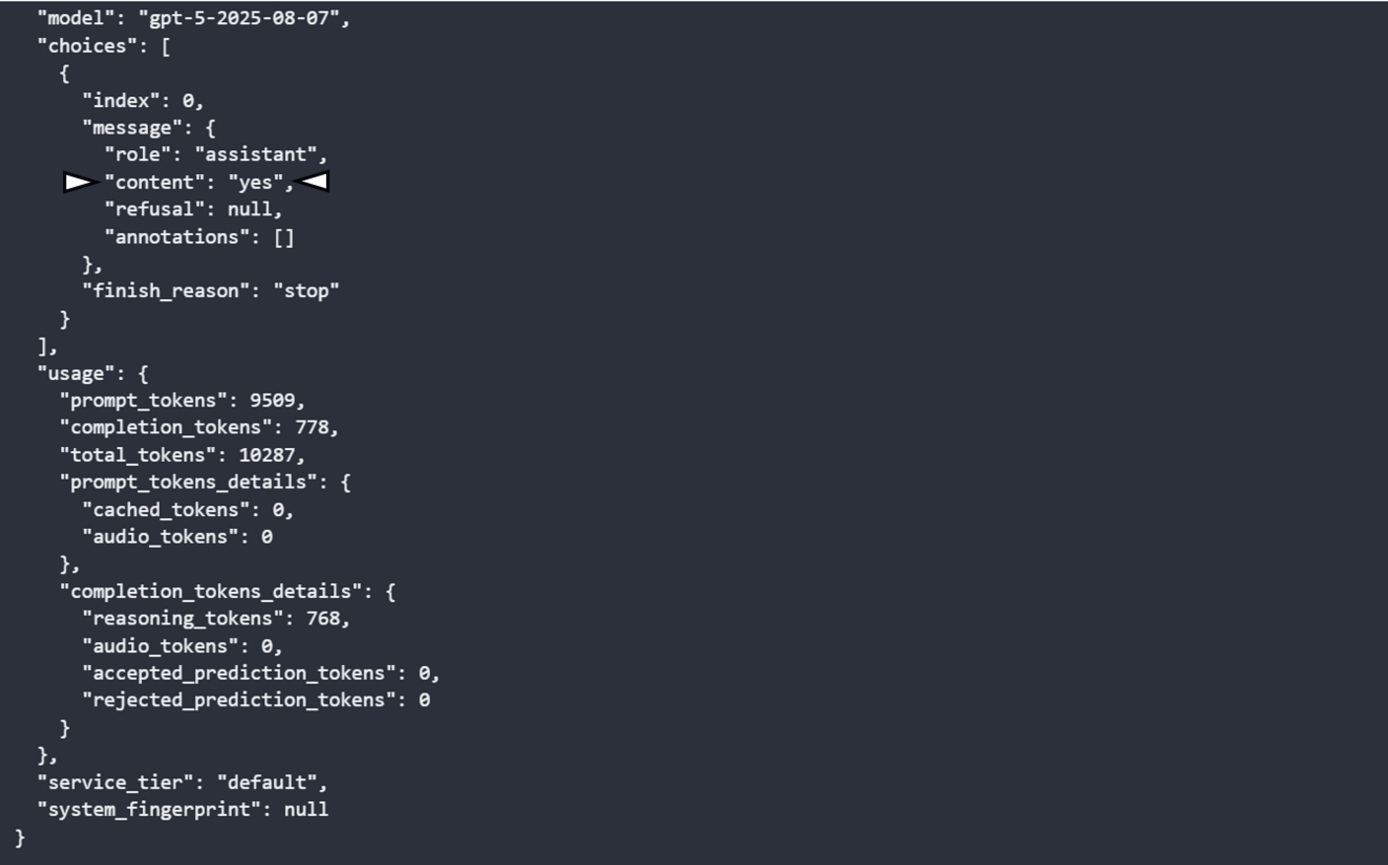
Example of OpenAI API response for the MRI slices input. This OpenAI API response showed that the gpt-5-2025-08-07 model successfully processed the prompt to analyze the input MRI slices and returned a simple reply ("yes") as shown at the "content" field (arrowhead). The detailed token usage is about 9,500 tokens for the input and 778 tokens for the completion, most of which were internal reasoning tokens. The request used the default service tier and encountered no errors or refusals. API: application programming interface; MRI: magnetic resonance imaging
